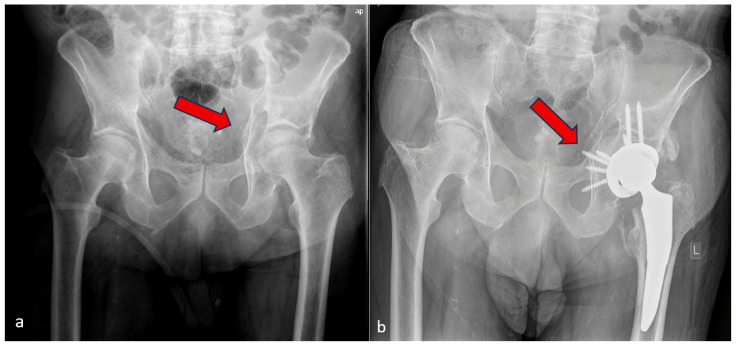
Acetabular fracture with intact anterior and posterior columns: (a) pre-operative radiograph showing quadrilateral plate fracture (red arrow), (b) one-year post-operative radiograph showing isolated THA augmented with femoral head autograft (cotyloidoplasty—red arrow).
